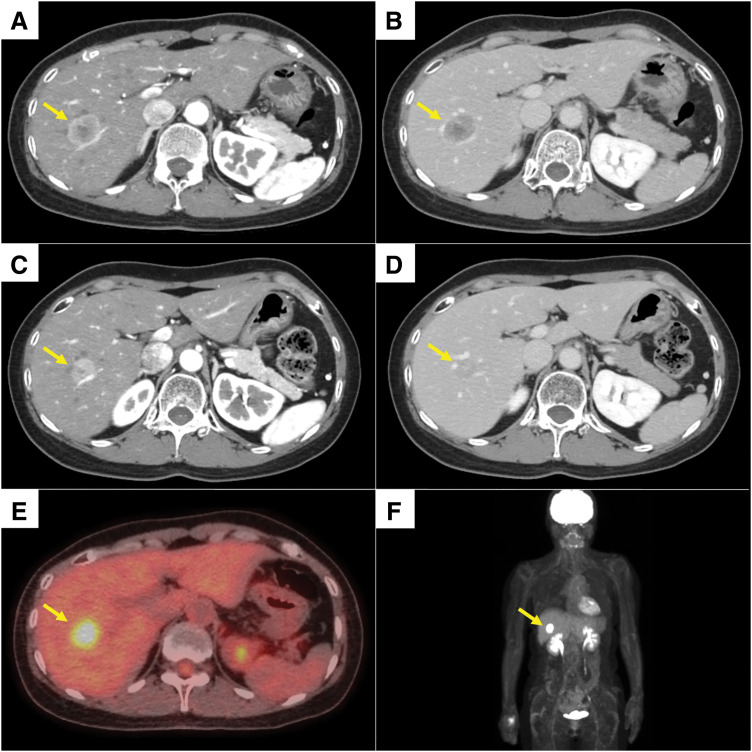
Contrast-enhanced CT and FDG-PET/CT images of the liver metastasis. The yellow arrows indicate the liver metastasis. (A) Arterial phase image at recurrence demonstrating a hyperenhancing lesion in segments 5 and 6 of the liver. (B) Delayed phase image showing relative hypoattenuation with peripheral enhancement. (C) Arterial phase image obtained after approximately 1 year of systemic therapy, demonstrating tumor shrinkage and reduced enhancement. (D) Delayed phase image after systemic therapy showing decreased lesion conspicuity compared with pretreatment imaging. (E) FDG-PET/CT image obtained after approximately 1 year of systemic therapy, demonstrating FDG uptake corresponding to the liver lesion (SUVmax: early 4.5, delayed 5.5). (F) MIP image showing no evidence of extrahepatic metastases; mild uptake in the right palm was considered physiological. At recurrence, the longest diameter of the liver metastasis was 28.5 mm. After approximately 1 year of systemic therapy, the lesion decreased in size to a longest diameter of 21.8 mm, corresponding to a tumor size reduction of 23.5% and classified as stable disease according to RECIST version 1.1. In both the recurrent and post-treatment (preoperative) images, the lesion demonstrated arterial phase hyperenhancement followed by relative washout in the delayed phase. FDG, fluorodeoxyglucose; MIP, maximum intensity projection; RECIST, Response Evaluation Criteria in Solid Tumors; SUVmax, maximum standardized uptake value
